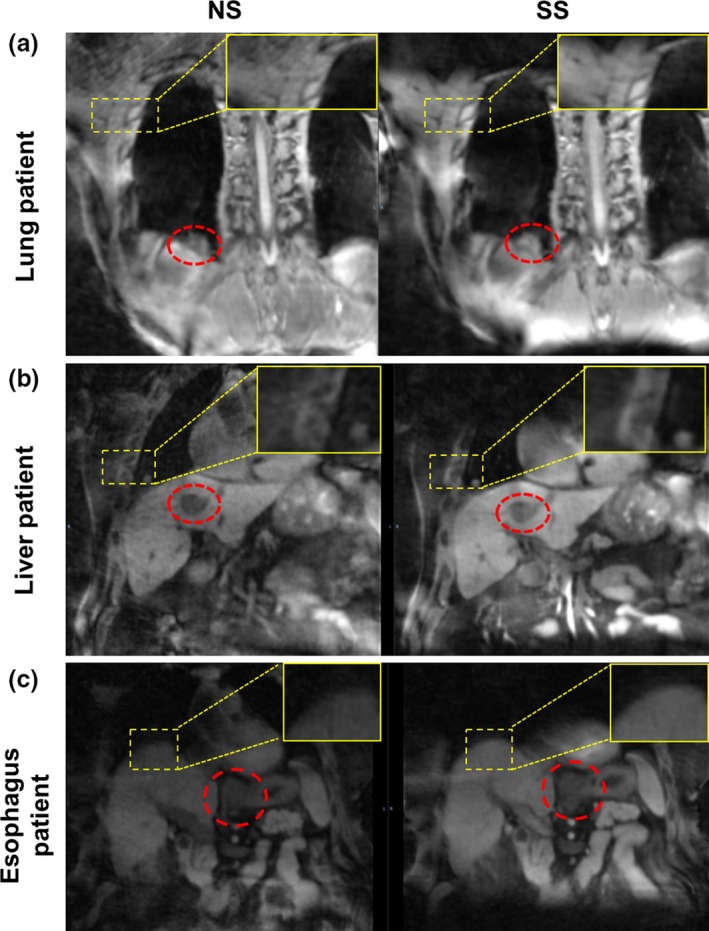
Image quality comparison between non‐selective (NS)‐4D‐MRI and slab‐selective (SS)‐4D‐MRI approaches in a (a) non‐small cell lung cancer patient with image score of 2 for SS and 1 for NS, (b) liver cancer patient with image score of 2 for SS and 1 for NS, and (c) esophagus cancer patient with image score of 3 for SS and 1 for NS. Red circles denote the tumor region in that particular patient.
